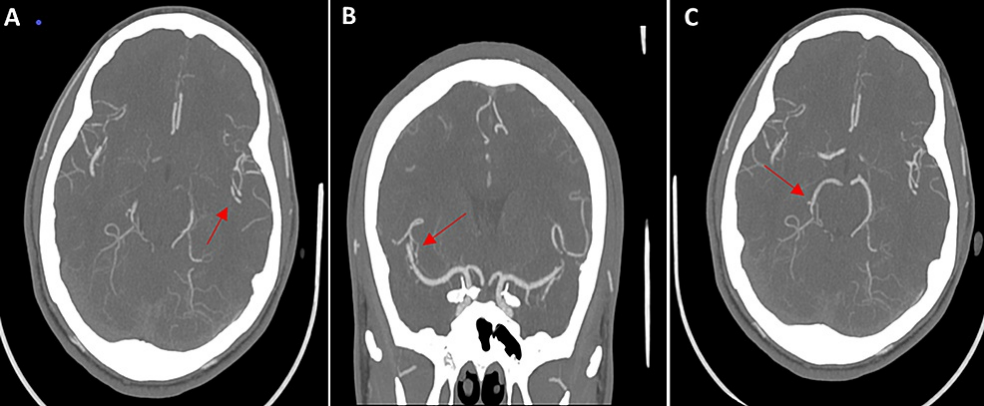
(A) Axial cut, CT angiogram of the head demonstrating focal areas of stenosis involving the left inferior M2 middle cerebral artery (MCA). (B) Coronal cut, CT angiogram of the head demonstrating focal areas of stenosis involving the right superior M2 MCA. (C) Axial cut, CT angiogram of the head demonstrating focal areas of stenosis involving the right P2 posterior cerebral artery.
